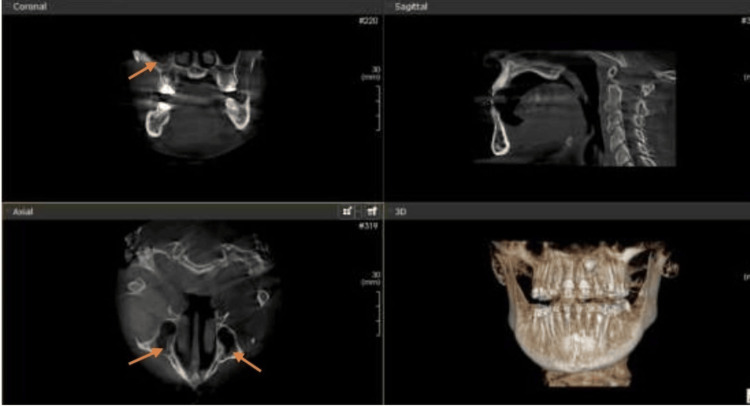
Maxillary sinusitis (orange arrows in both coronal and axial sections) Maxillary sinusitis in coronal and axial CBCT sections of an orthodontic patient. CBCT: Cone-beam computed tomography
